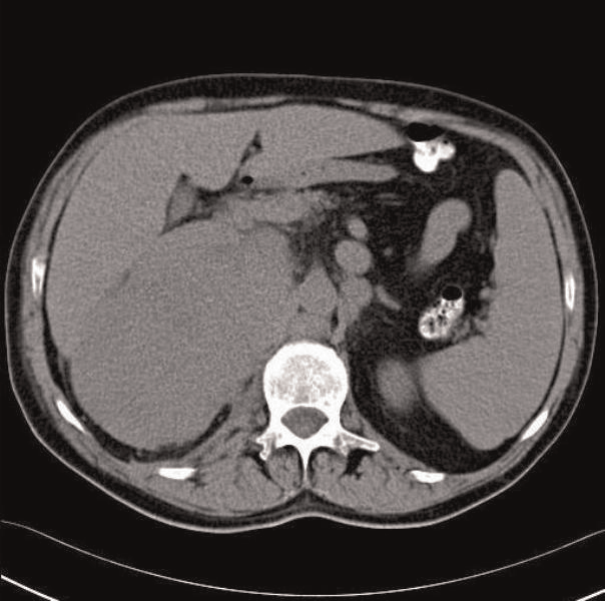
Abdominal CT scan (native) image showing hypodense renal mass involving the right kidney with perinephric extension.
